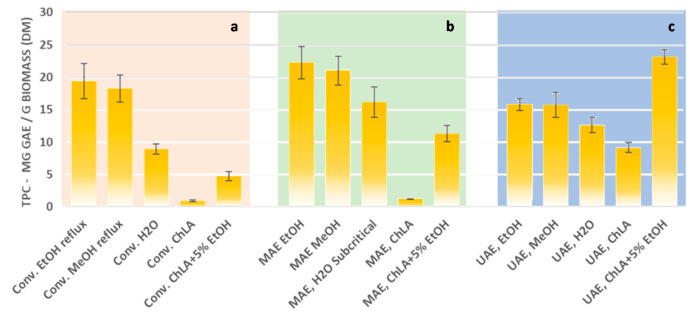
Comparative extractions of Potato-Peel (PP) under conventional (a) and non-conventional technologies ((b) utilises microwave (MW), (c) ultrasound (US)) exploiting sustainable solvents.
